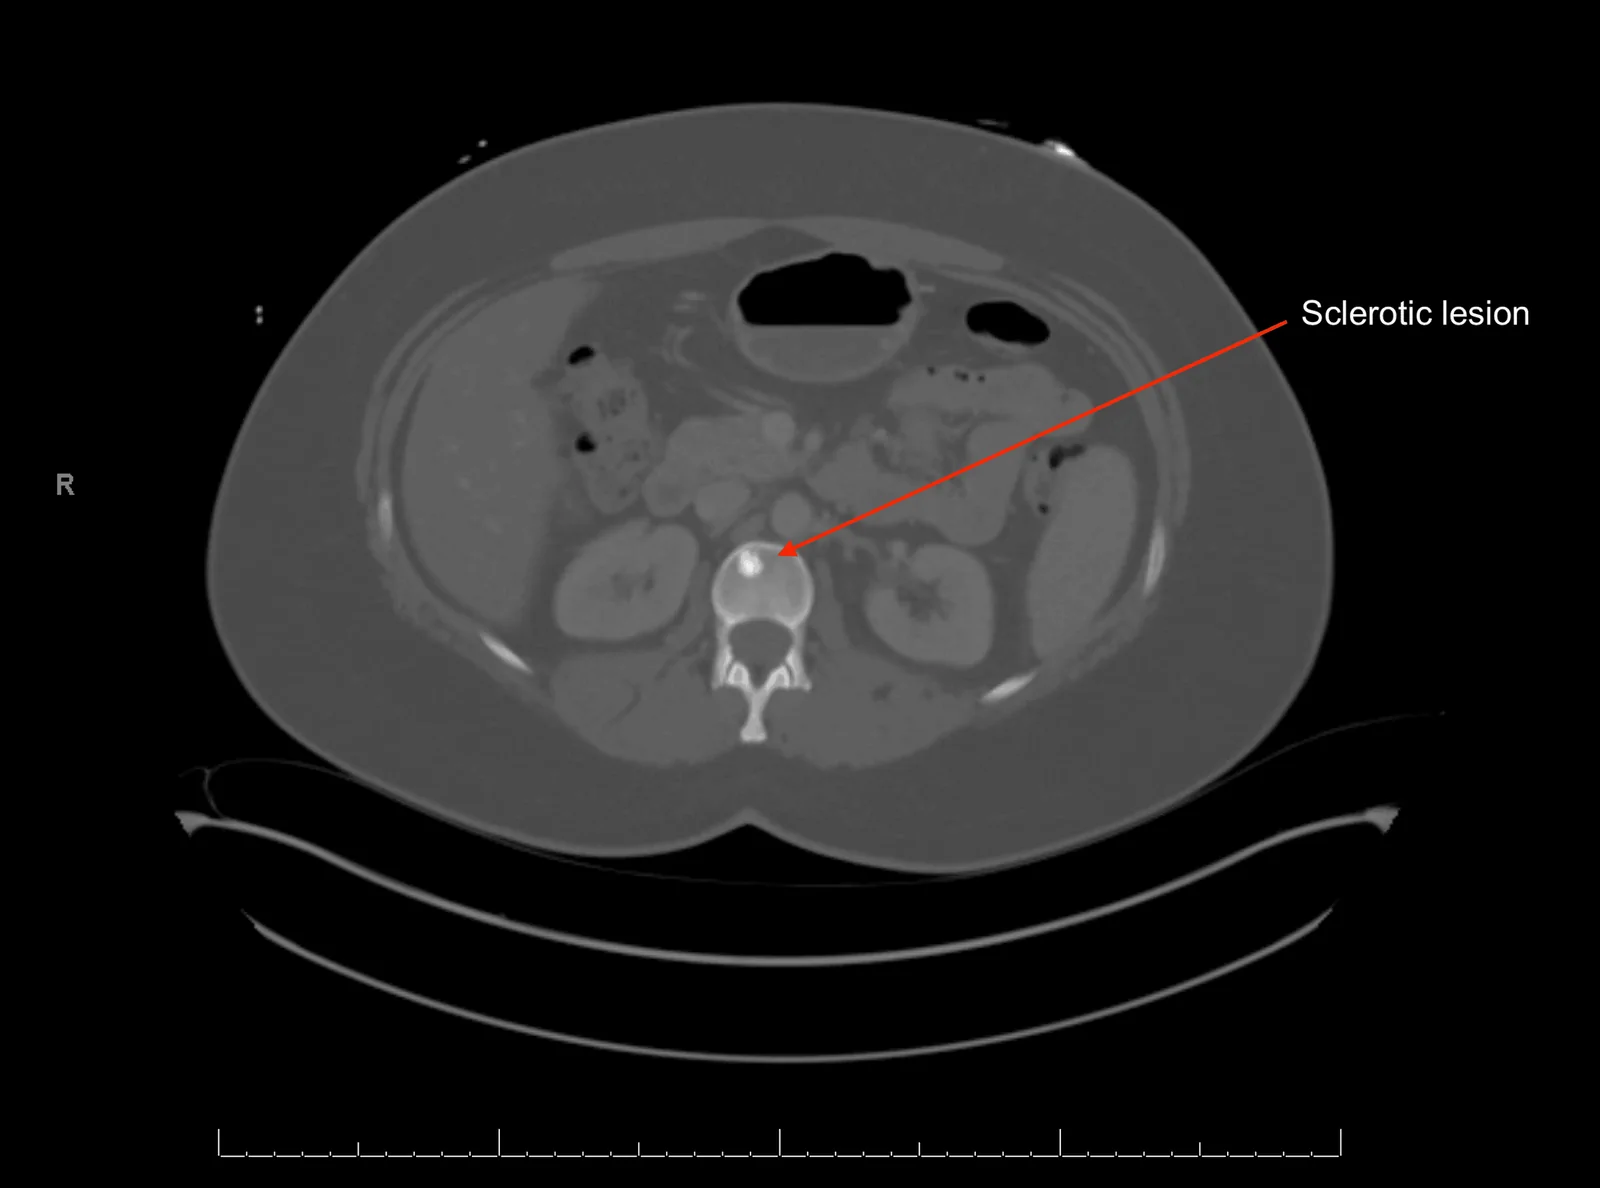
Computed tomography (CT) chest demonstrating a small focal sclerotic focus seen at L2 vertebral body measuring up to 1.1 cm in size
